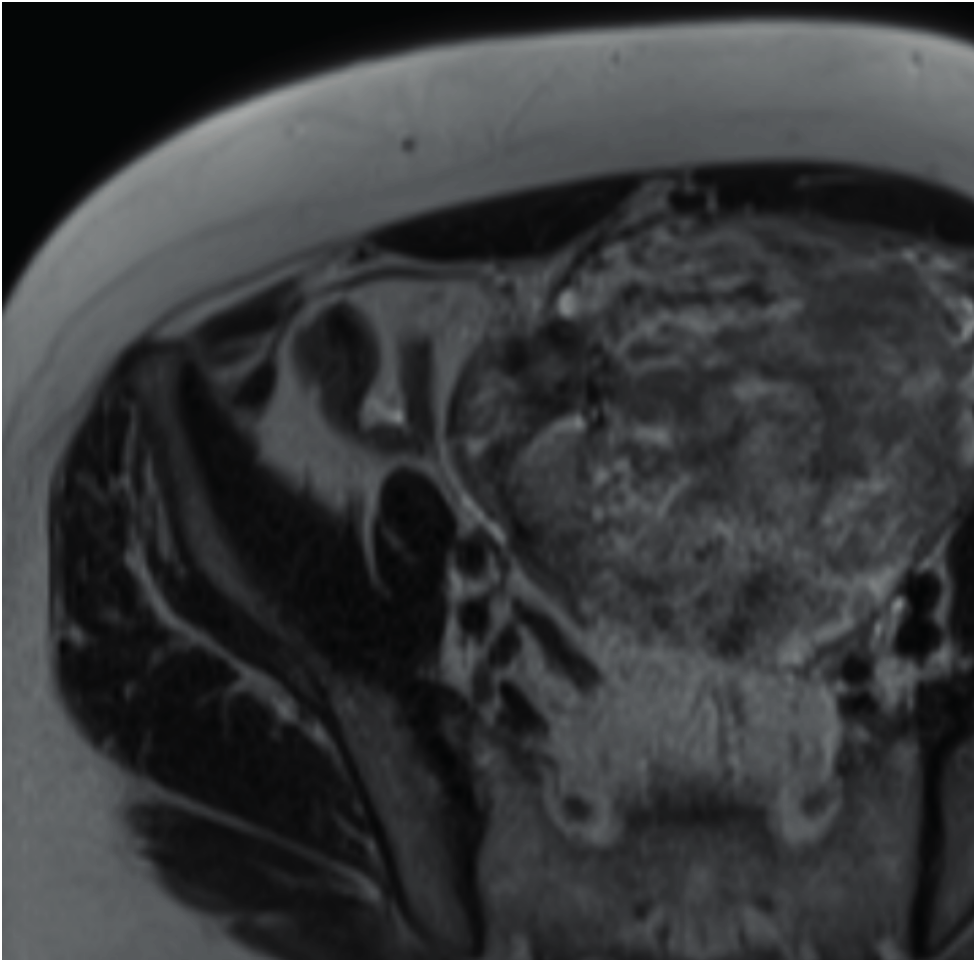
Transversal MRI image of the pelvis showing the extensiveness of the parasitic leiomyoma.
